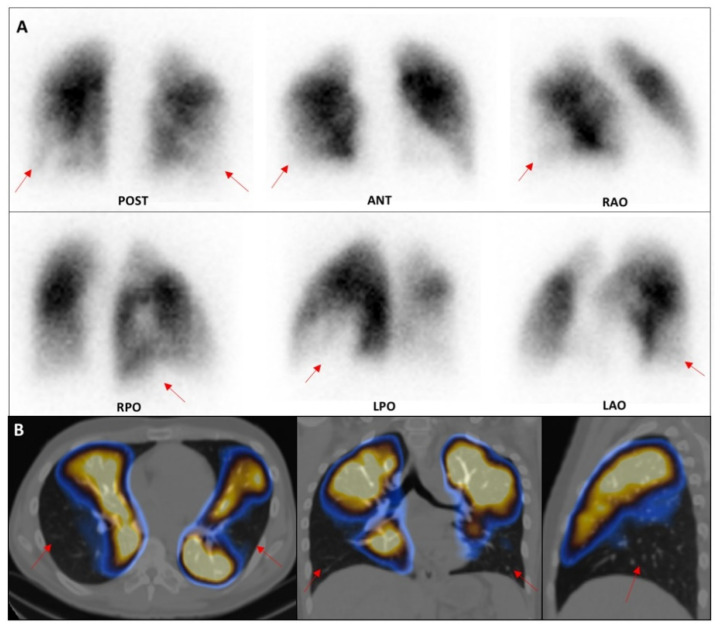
Lung perfusion scintigraphy performed for the suspicion of APE in a 32-year-old patient affected by a genetic rare disease (MTHFR mutation), who presented severe dyspnoea, hypoxia/hypocapnia at arterial blood gas analysis, increased d-dimer values (3253 ug/L, n.v. < 500), and referred allergic diathesis. Planar (A) and SPECT/CT (B) acquisitions demonstrated multiple wide wedge-shaped segmental perfusion defects in both lower lobes, thus confirming the suspicion of APE. Consequently, the patient was immediately referred to the intensive care unit for prompt therapeutic support. POST: posterior; ANT: anterior; RPO: right posterior oblique; RAO: right anterior oblique; LPO: left posterior oblique; LAO: left anterior oblique.
